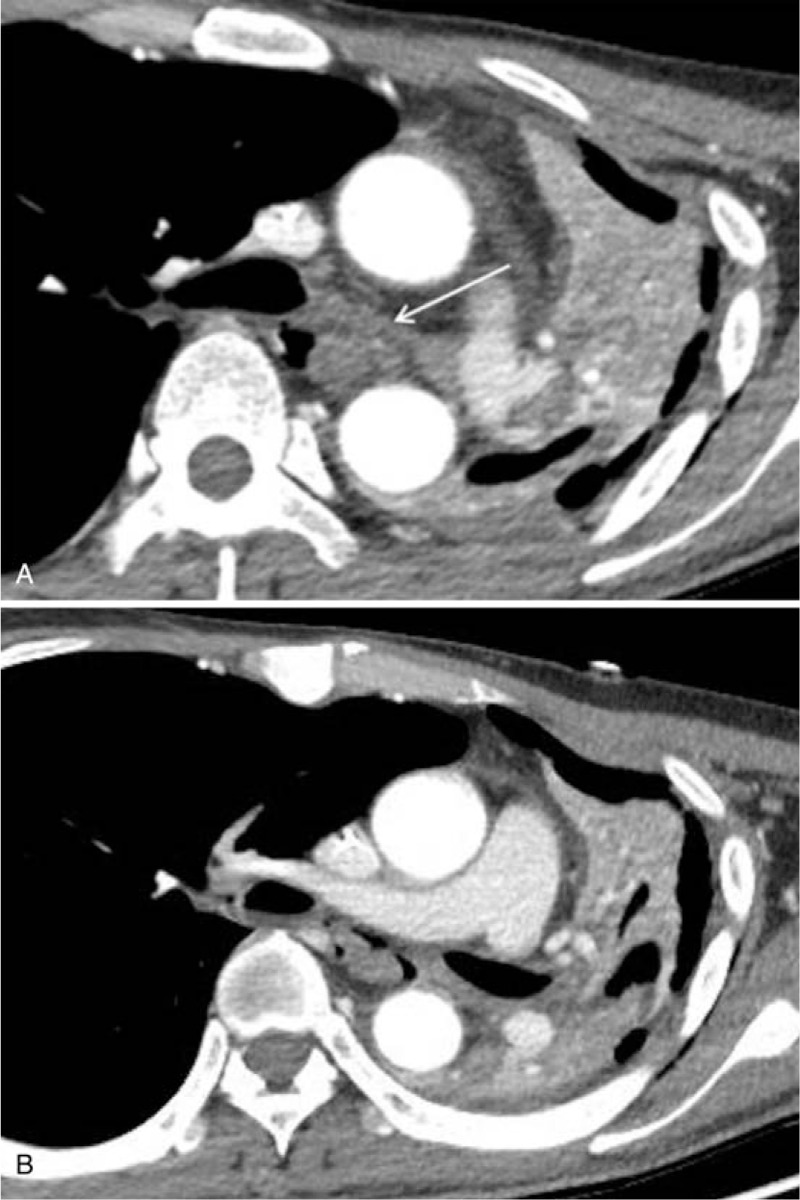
Chest CT findings of late airway complication 6 months post acid ingestion. A, B. Progressive narrowing of the left main bronchus (arrow) is shown on chest CT; resultant total collapse of the left lung is also seen.
